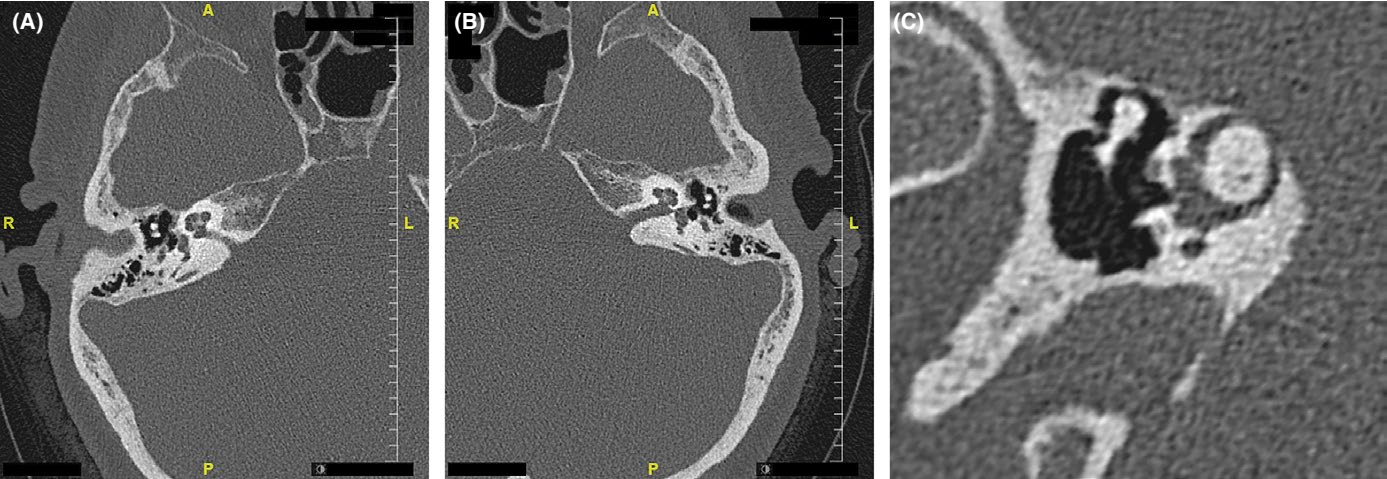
An axial right (A) and left (B) computed tomography scan of the patient described in the case presentation. Bilateral otosclerosis is seen. A reconstructed Pöschl's view of the right temporal bone (C). A dehiscence of the right superior semicircular canal is easily seen
